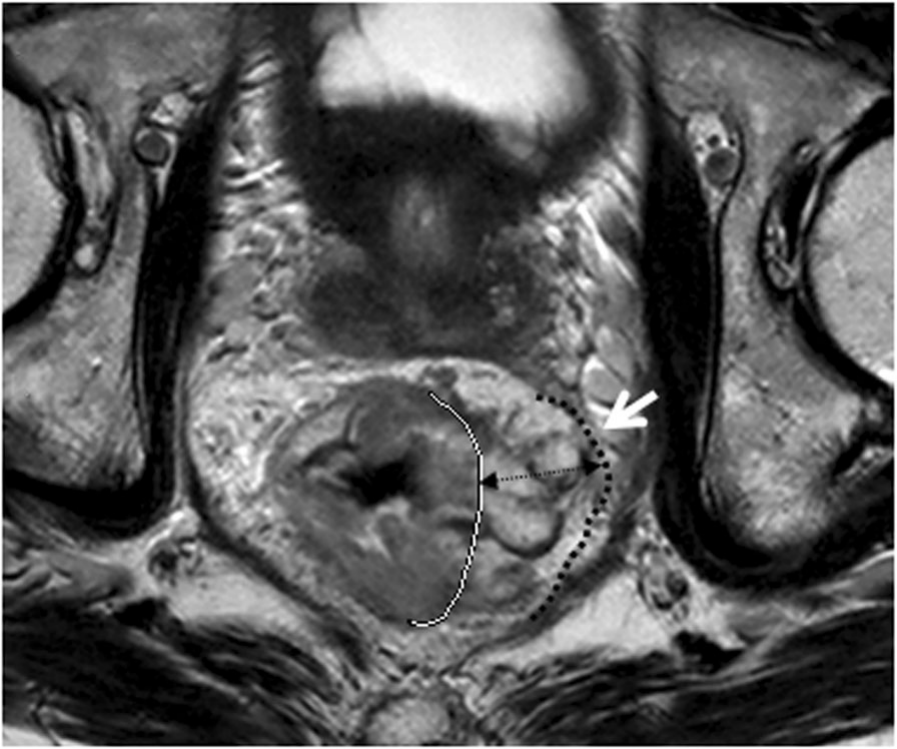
Oblique axial T2-weighted imaging of a rectal mucinous adenocarcinoma (intermediate to high signal intensity) presenting as bulky mass showed significant tumor infiltration beyond the muscularis propria; the maximal extramural depth (MEMD, double-headed arrow) was over 10 mm. Meanwhile, the invasive border of rectal mass bordering the mesorectal fascia (white arrow) which leaded to a CRM of 0 mm. White line = muscularis propria border. Black dashed line = the mesorectal fascia
